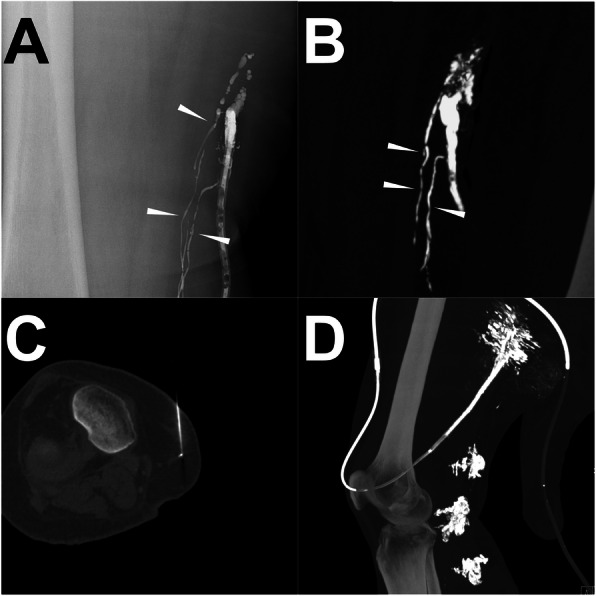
A patient underwent ALVE procedure after post-LAG CT. Note: Conventional iodized oil-based LAG showed the definite extravasation from three afferent LVs into the drainage (a). A similar demonstration was observed from the sagittal MIP images of post-LAG CT (b). The clear afferent LVs are shown (white arrowheads). The afferent LVs were punctured using the 21-G needles under the CT guidance penetrating the anterior and posterior walls (c). After the “cutting-off” embolization by using the histoacryl/lipiodol mixture (2:3) (d). The LF ceased immediately. Abbreviations: ALVE – afferent lymphatic vessel embolization; post-LAG CT – post-lymphangiographic computed tomography; LAG – lymphangiography; LVs – lymphatic vessels; LF – lymphatic fistula
